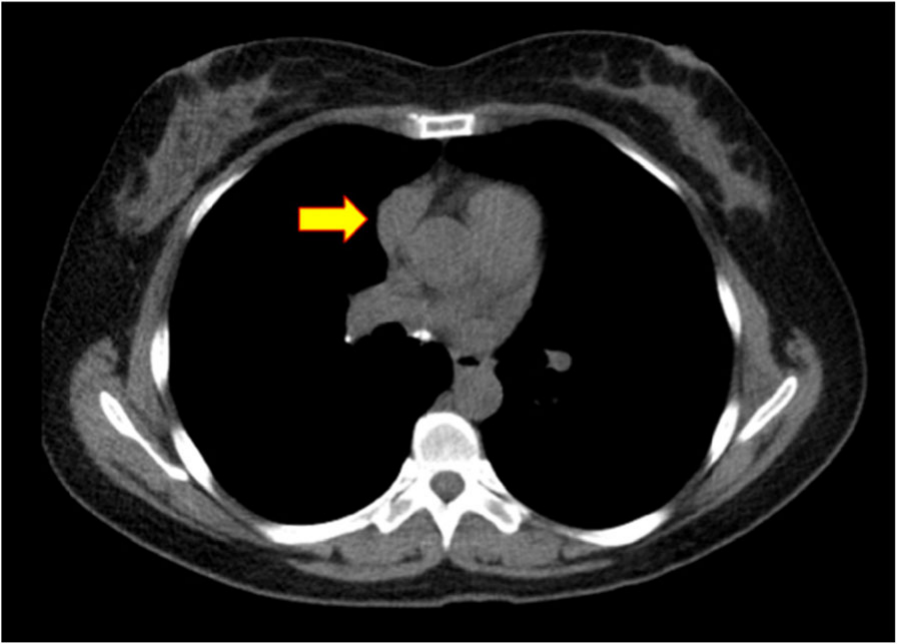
Preoperative CT of 3.5 cm × 2 cm anterior mediastinal mass along the right heart border and phrenic nerve
